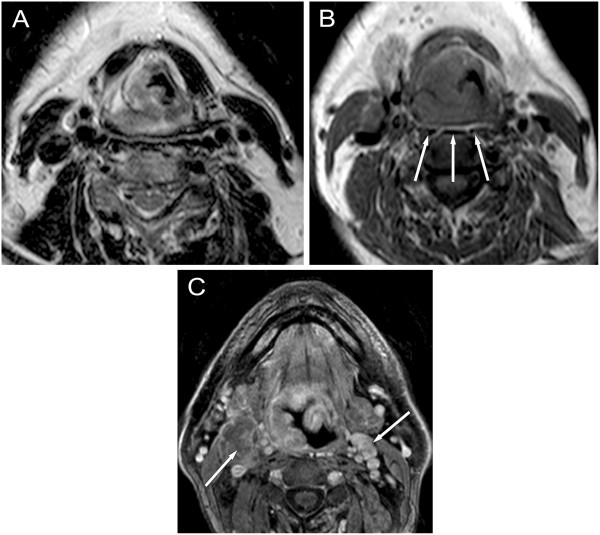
Hypopharyngeal cancer, stage T4aN2c. A: axial T2-weighted, B: axial non-enhanced T1-weighted MR images show cancer of the hypopharynx involving the dorsal and right lateral pharyngeal wall, avoiding the retropharyngeal space. Note the intact prevertebral fat, clearly obtainable on T1-weighted image (arrow). C: axial post-contrast T1-weighted MR image with fat suppression at the level of the tongue base, tumour infiltrates the tongue base crossing the midline to the opposite side. Metastatic lymph nodes are present bilaterally (arrows).
